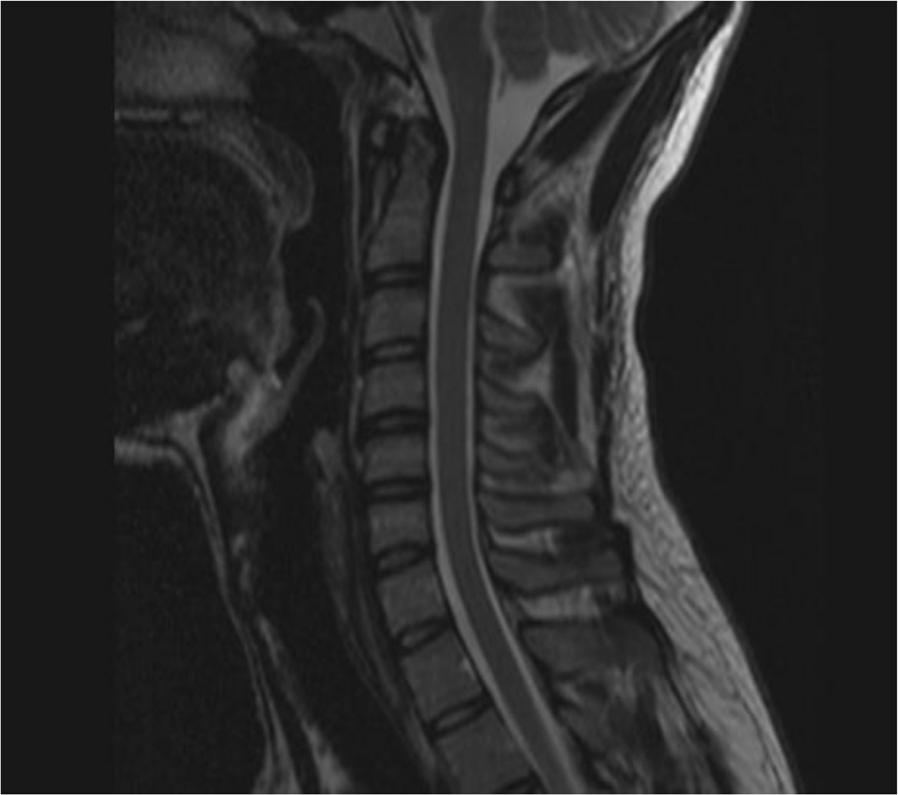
Cervical spine magnetic resonance imaging (1.5 Tesla). Sagittal T2 section, demonstrating normal spinal cord and dural canal in the neutral position
